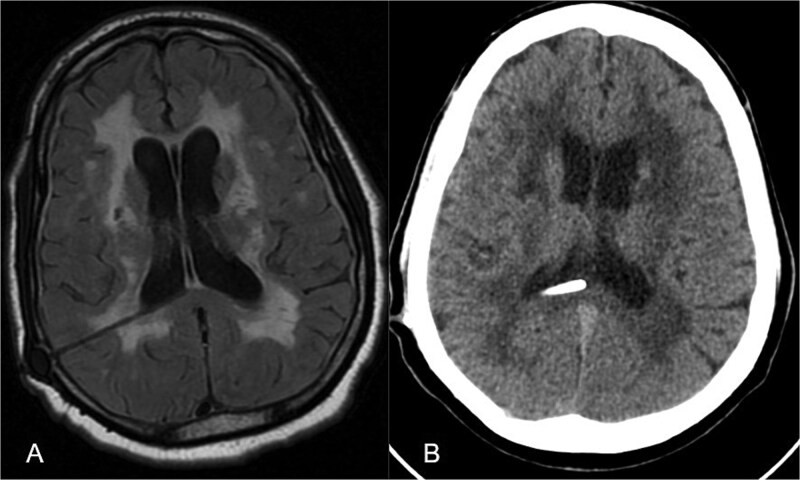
Post-operative imaging following ventriculoperitoneal shunt placement. (A) Axial T2-FLAIR MRI at 2 months demonstrating reduction in transependymal oedema. (B) Non-contrast CT at 6 months demonstrating decreased ventriculomegaly with ventriculoperitoneal shunt catheter in situ.
